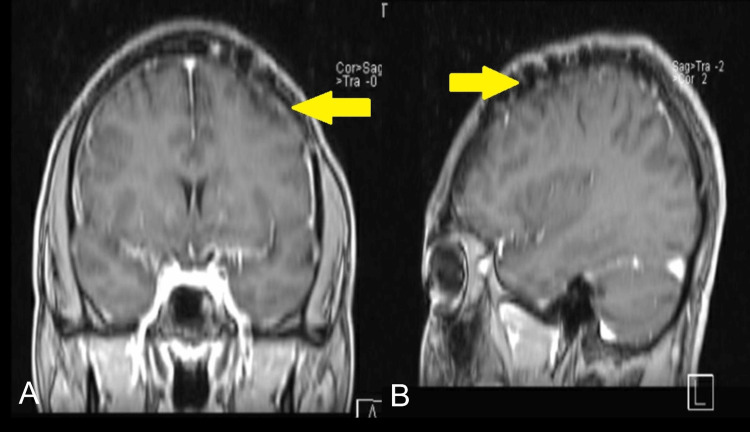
Brain MRI Image at one-year follow-up. (A) Post-contrast coronal T1-weighted brain MRI image at one-year follow-up and (B) post-contrast sagittal T1-weighted brain MRI image at one-year follow-up.
